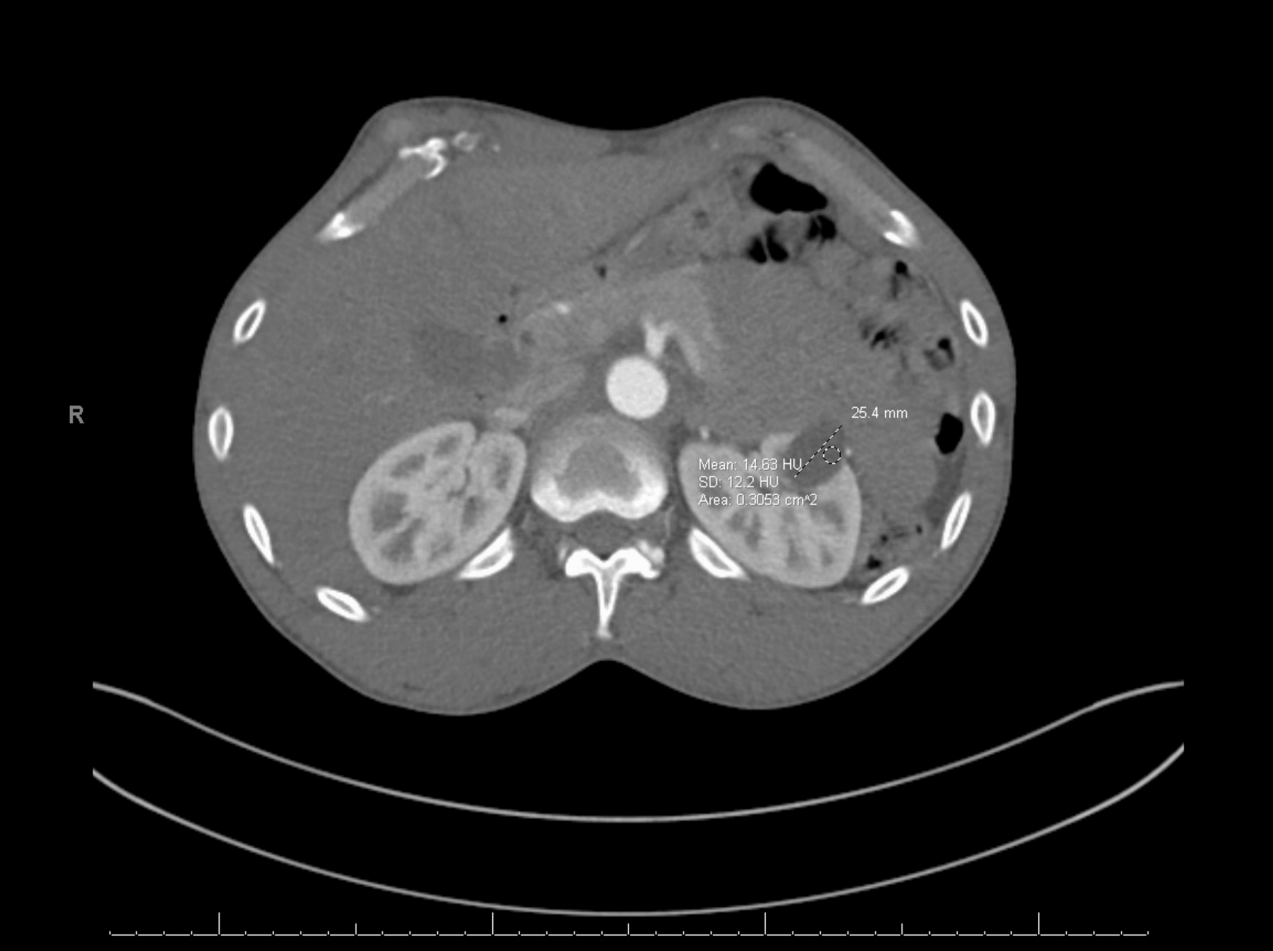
CT angiogram of the aorta and bilateral iliofemoral arterial runoff, which showed an indeterminate low-density lesion in the left kidney measuring 2.5 cm, indicative of a benign left renal cyst CT: computed tomography
